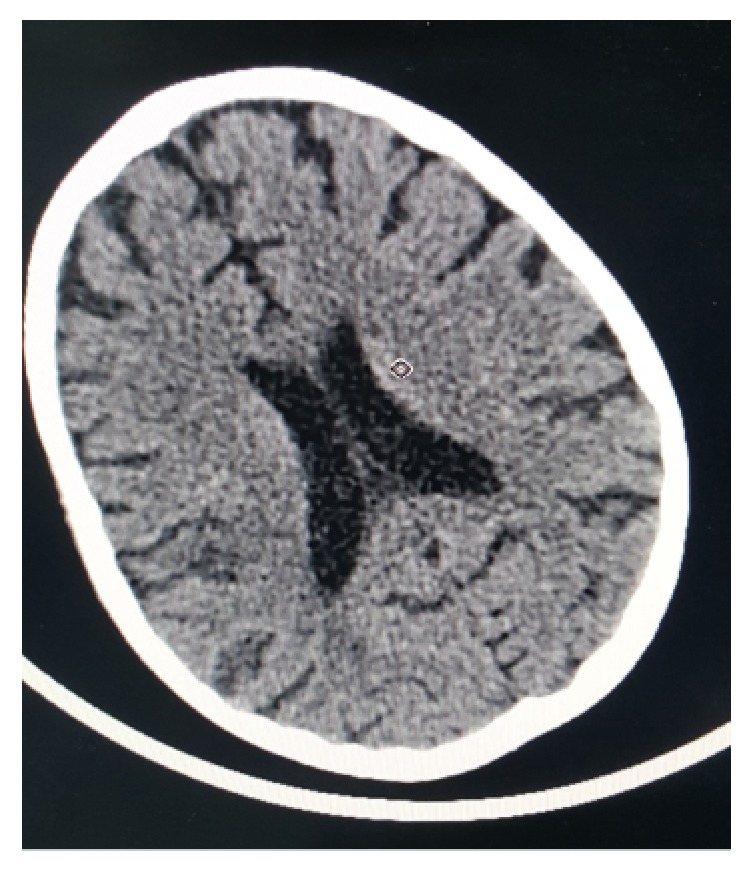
Patient CT scan showing showed small hypodensity foci situated in the cortical and subcortical area in right partial lobe.
